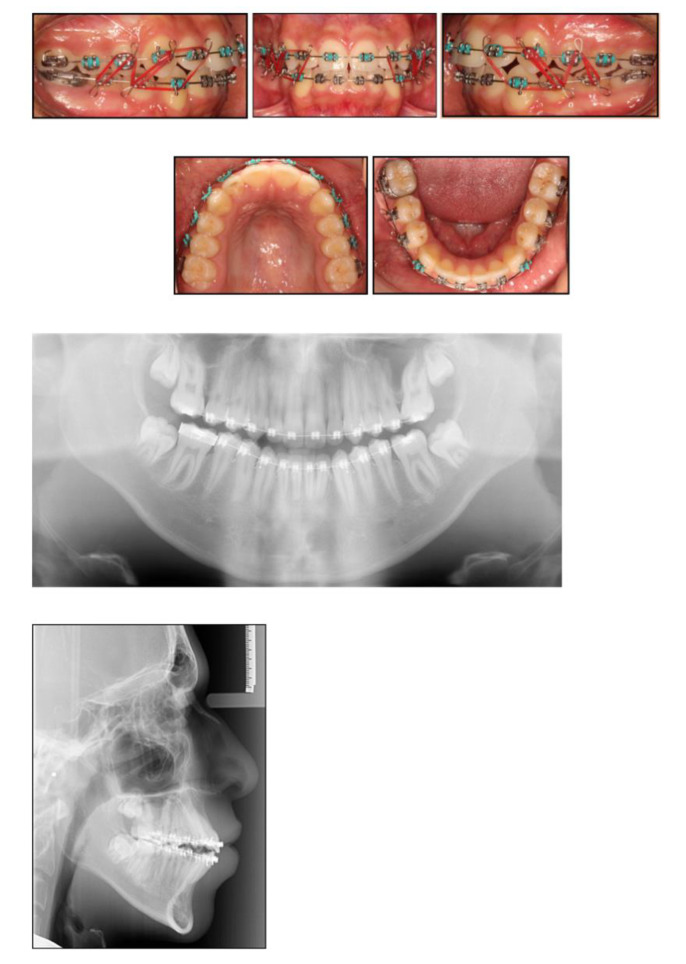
Near-end-of-treatment stage following space closure. Near-end-of-treatment radiographs revealed favourable third molar positioning. Intermaxillary elastics with a vertical vector were utilised to actively enhance interdigitation.
